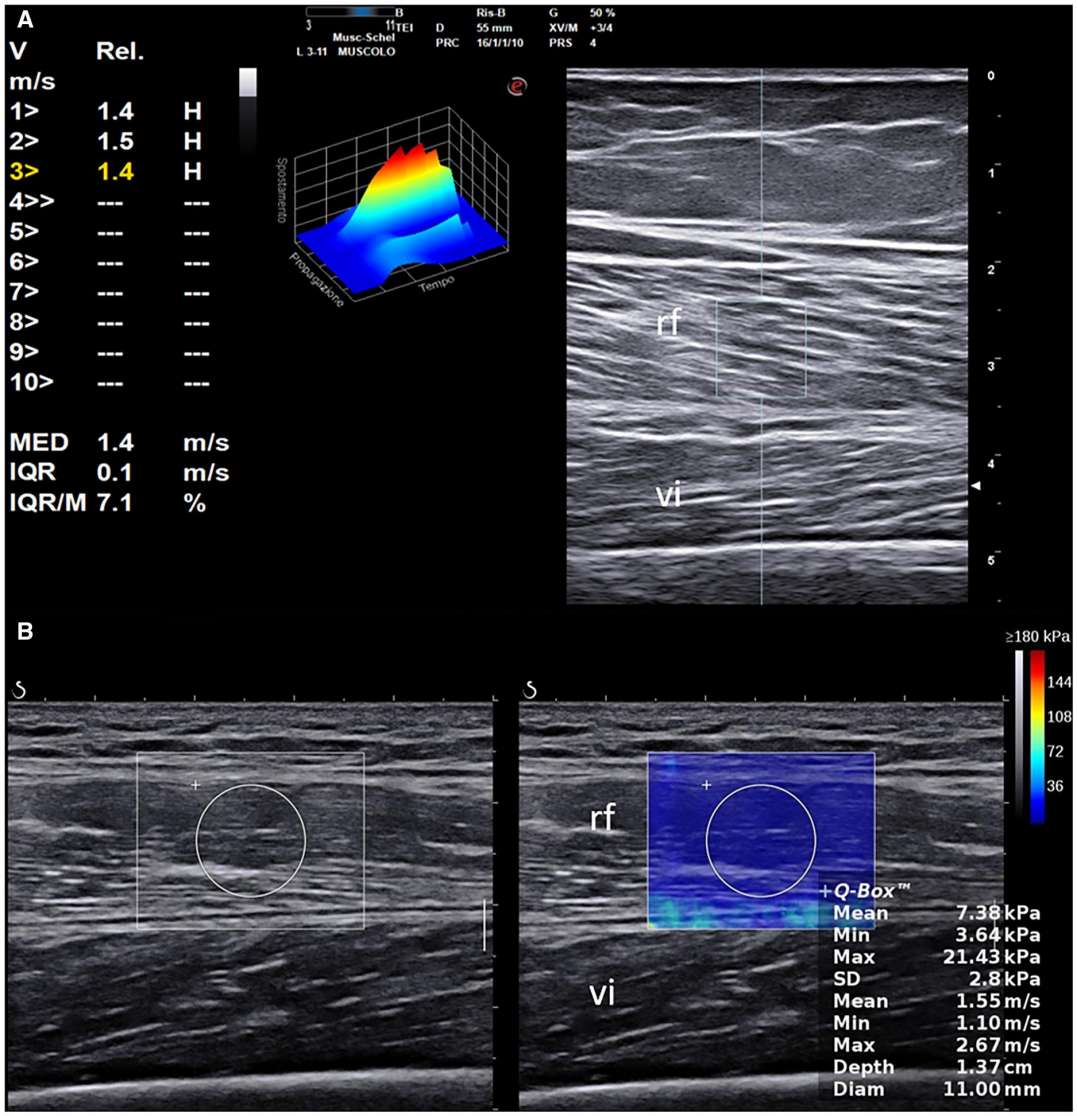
Shear-wave elastography measurements in SSc patients. Ultrasound images of the rectus femoris muscle in two patients with systemic sclerosis (SSc). (A) Point shear-wave elastography (pSWE) acquisition in a patient with diffusely increased muscle echogenicity. (B) 2D shear-wave elastography (2D SWE) in a different patient, with mildly reduced muscle thickness and increased echogenicity, particularly in the lower rectus femoris. Measurement regions are indicated: a rectangle for pSWE and a rounded area within a rectangle for 2D SWE. Results are in metres per second. Labels include rf (rectus femoris) and vi (vastus intermedius)
